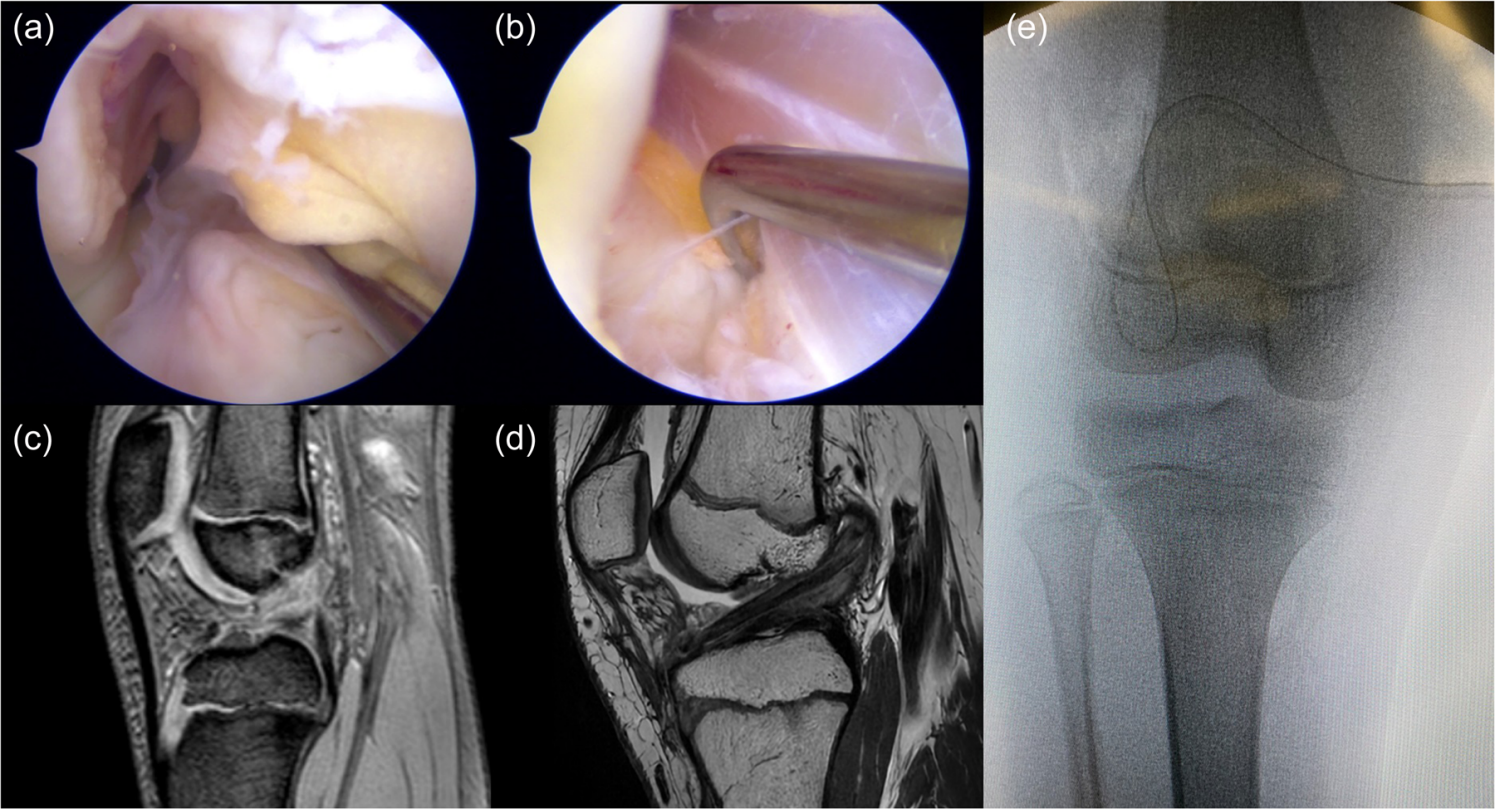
A male football player with chronological age of 11.2 years and skeletal age of 11 years was treated with extra‐physeal over‐the‐top ACL reconstruction. Arthroscopic vision with the empty notch due to ACL injury (a) and after reconstruction with hamstring graft under the intermeniscal ligament (b). Sagittal pre‐operative MRI shows the absence of the ACL (c) and the 4‐month MRI shows the graft with a good ipointense signal and the extra‐physeal oblique course, with no tibial tunnel (d). Antero‐posterior radiograph shows the absence of tunnels or hardware (e). ACL, anterior cruciate ligament; MRI, magnetic resonance imaging.
